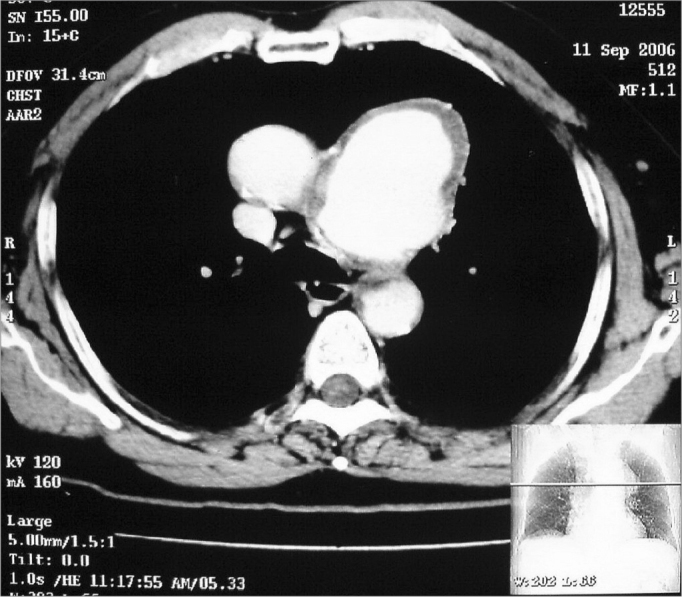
Contrast enhanced CT scan chest, axial view showing large, lobulated, enhancing saccular aneurysm arising from the arch of aorta with peripheral thrombus.
